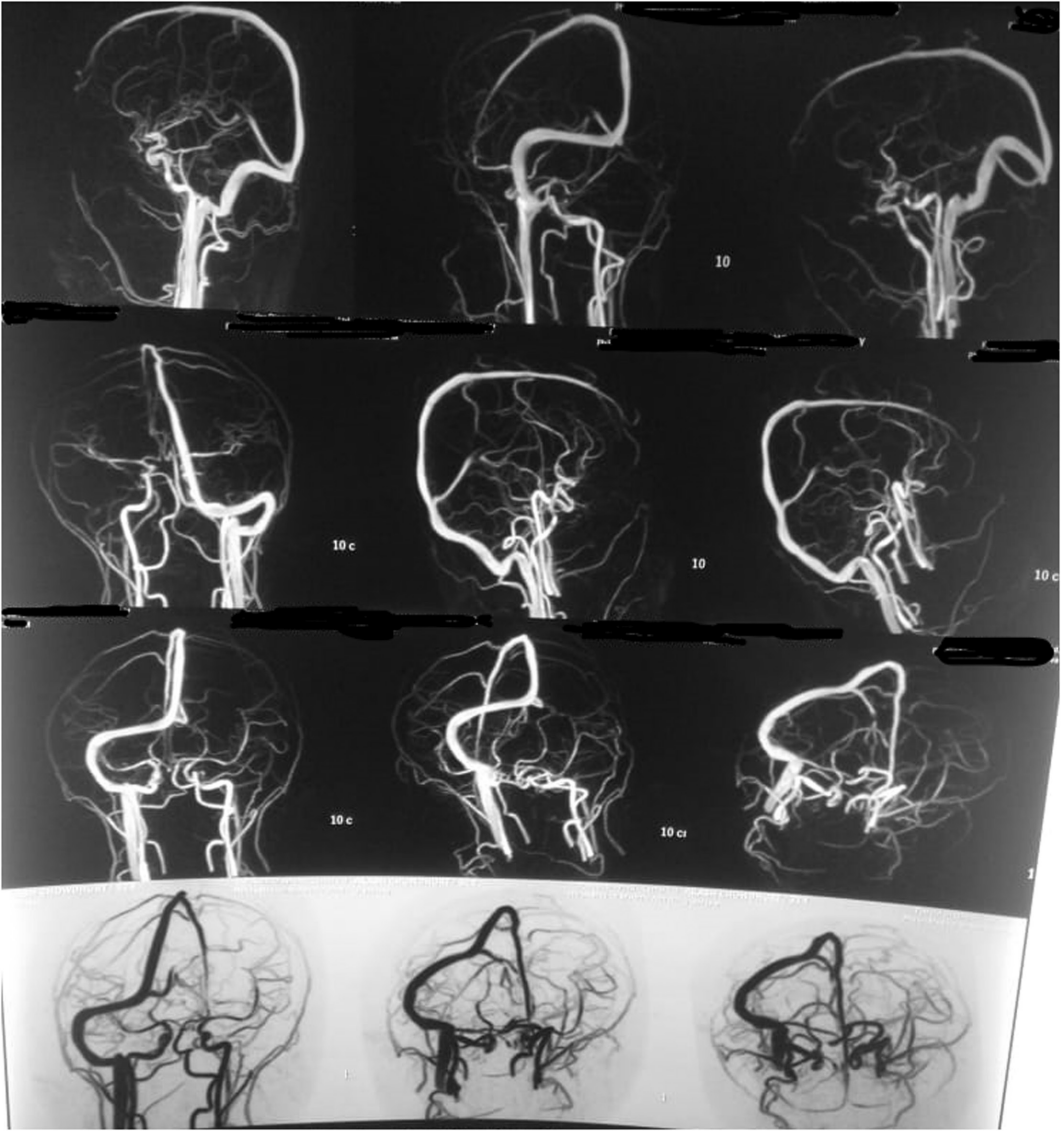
Magnetic Resonance Venography (MRV): Sagittal view of collateral system (dynamic magnetic resonance image) of the case: Revealed the jugular venous system of the left side is not visualized and the right side jugular venous system is prominent and deep veins and dural venous sinuses appear normal which suggestive of congenital agenesis of jugular venous system of left side.
